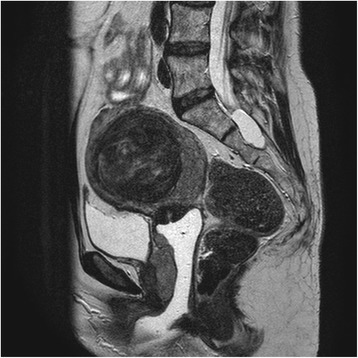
MRI showing a leiomyoma and posteriorly a mass that extends to the uterine isthmus-cervix juncture
